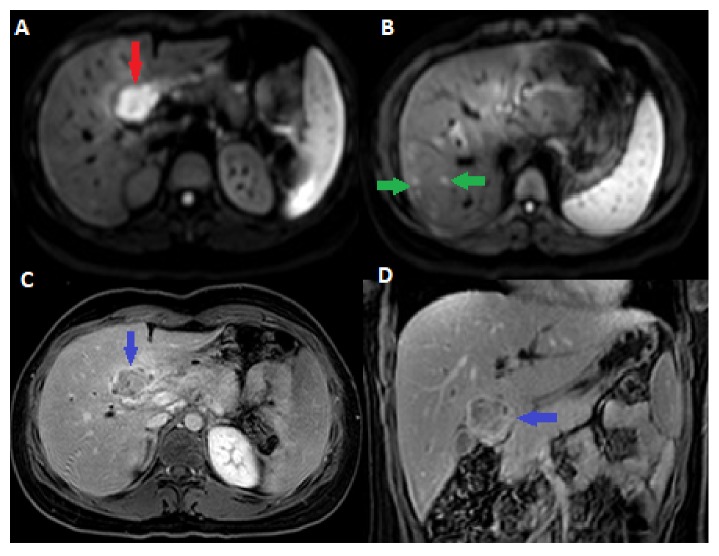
MRI of the liver axial diffusion-weighted (A, B) demonstrates a hyperintense mass in the region of the porta hepatis (red arrow) with a several hyperintense foci (green arrows). Axial postcontrast images (C, D) show heterogonous enhancement of porta hepatis mass (blue arrows).
